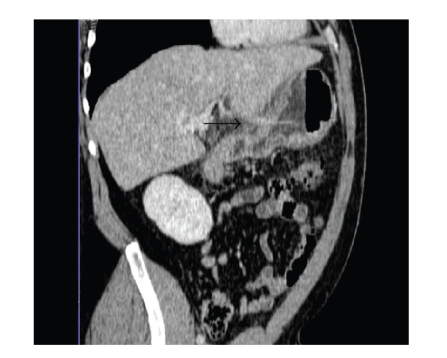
Computed tomography image with a pattern possibly revealing a toothpick
